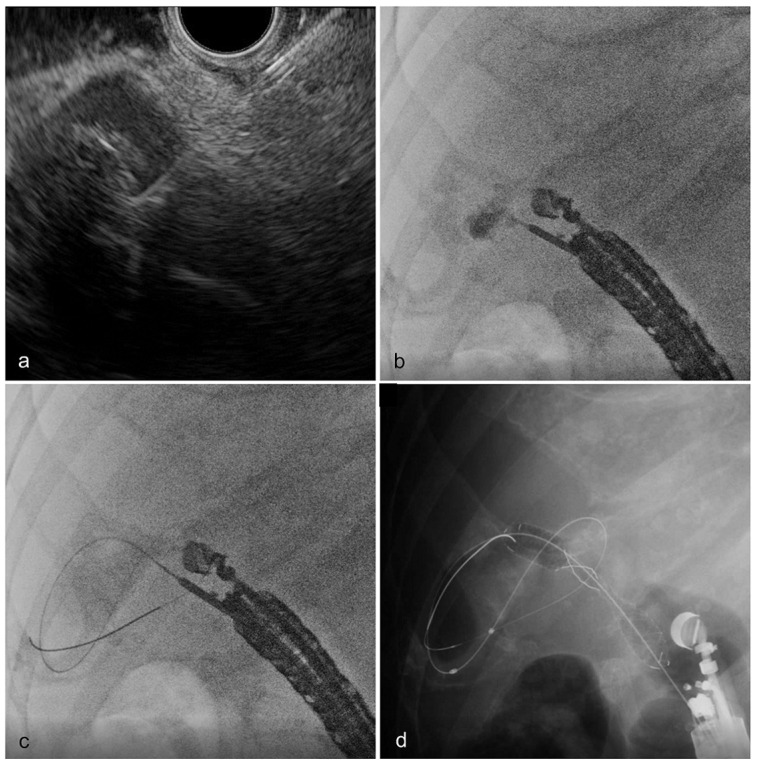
Techniques of endoscopic ultrasound-guided gallbladder drainage. (a) The gallbladder was detected using endoscopic ultrasound and punctured using a 19 G fine aspiration needle, while avoiding conspicuous blood vessels. (b) After adequate aspiration of infected bile, the contrast agent was administered through the needle. (c) After deep insertion of a guidewire into the gallbladder, the puncture tract was dilated using a dilator along the inserted guidewire. (d) A fully covered dumbbell-type metal stent was placed from the gallbladder to the gastrointestinal tract.
